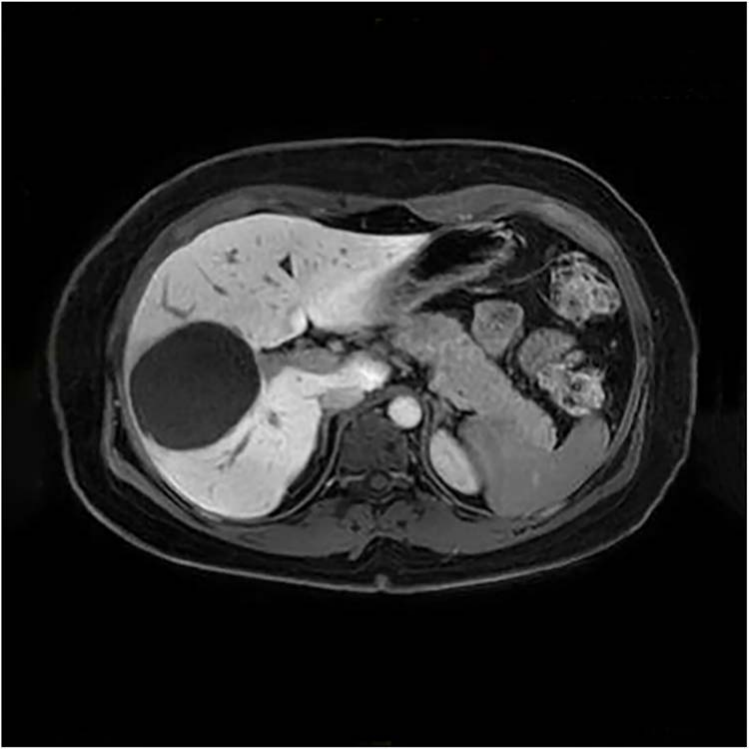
Evidence of a size-progressive septated liver cyst in the MRI.
